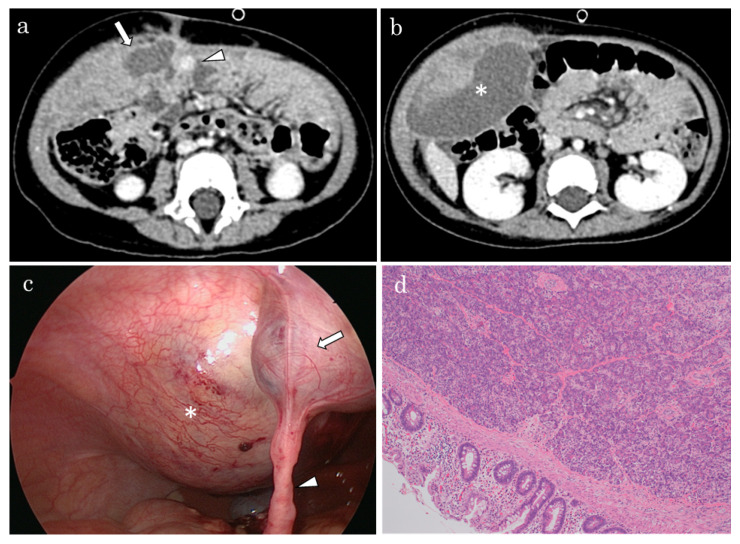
Contrast-enhanced computed tomography. (a) A 4 cm cystic lesion (arrow) located just beneath the umbilicus, containing an enhanced lesion of 1 cm in diameter (arrowhead). (b) On the immediate right side of this cyst, a 7 cm hematoma (*) is seen within the abdominal wall. (c) Laparoscopic observation reveals an omphalomesenteric cyst (arrow) beneath the umbilicus and a mesodiverticular band (arrowhead) connecting to the mesentery. These lesions are accompanied by a hematoma (*) extending to the right abdominal wall. (d) Pathological examination reveals that the omphalomesenteric cyst wall is lined with small intestinal and stomach mucosa, and the solid nodule contains pancreatic tissue mucosa.
